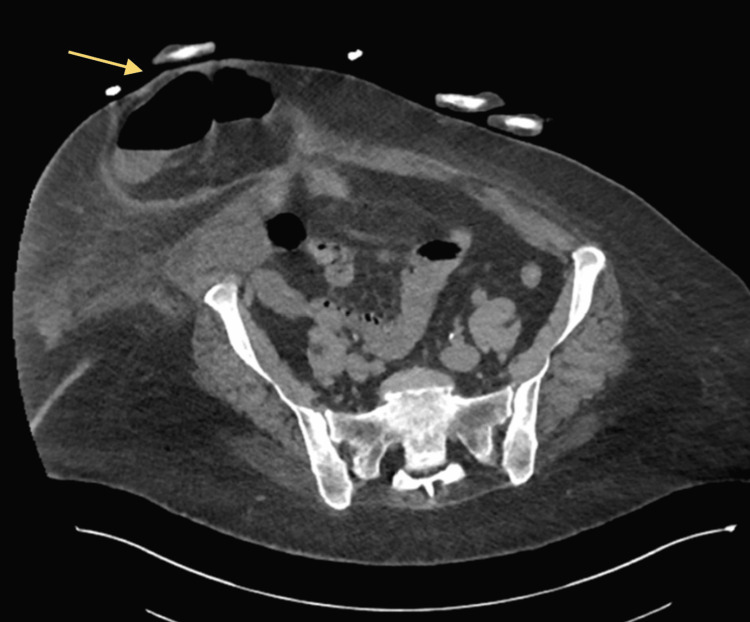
CT abdomen and pelvis: large paraumbilical hernia secondary with a neck of 3cm.
